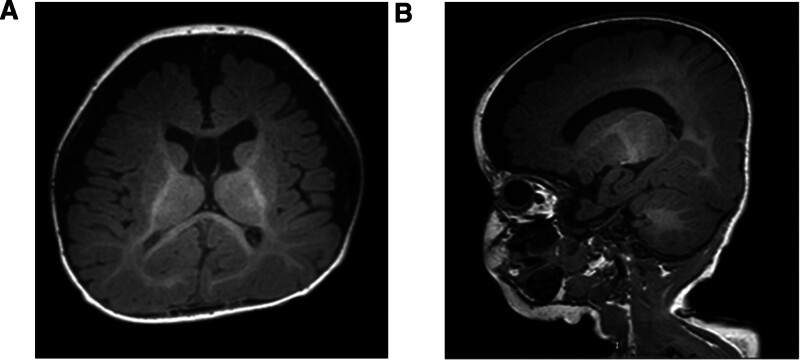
Magnetic resonance imaging of the pediatric proband. (A) Delayed myelination of white matter and significant widening of the cerebrospinal fluid space by axial T1-weighted brain MRI; (B) delayed myelination of white matter and apparent widening of the cerebrospinal fluid space by sagittal T1-weighted brain MRI. MRI = magnetic resonance imaging.
